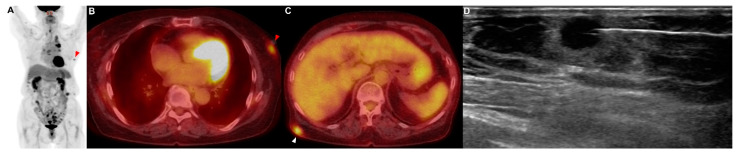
Patient 3: a 79-year-old woman diagnosed with lung adenocarcinoma with metastases in subcutaneous tissue. (A–C) The PET/CT showed a metastatic lesion (red arrowhead) in the left breast. I PET/CT showed a metastatic lesion (white arrowhead) in the subcutaneous layer of the middle part of the right back. (D) The core needle biopsy was performed on the lesion of the left breast.
